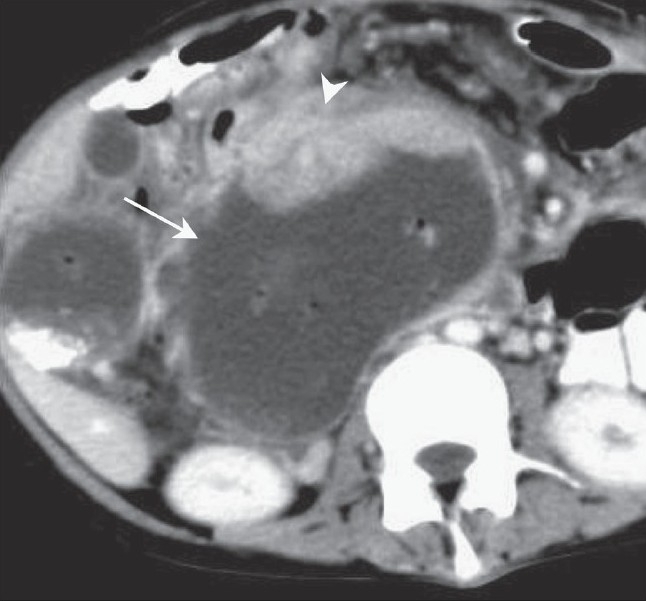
Contrast-enhanced axial CT scan through the inferior part of the cyst shows an enhancing, eccentric, lobulated mass (arrowhead) arising from the anterior wall of the cyst (arrow). Air and oral contrast within the cyst are noted
